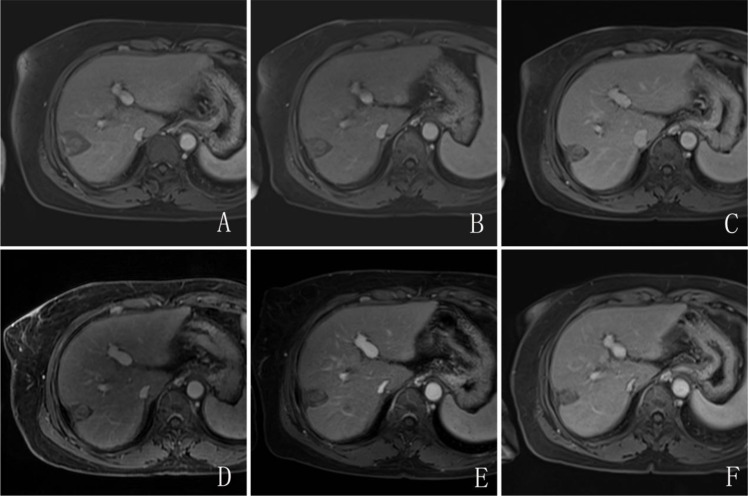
The same patient as in Figure 2. MRI re-examination at 1 month (A), 3 months (B), 6 months (C), 1 year (D), 2 years (E), and 3 years (F) after the combined transcatheter arterial chemoembolization and DynaCT-guided microwave ablation (TACE+MWA) procedure did not show obvious enhanced lesions.
